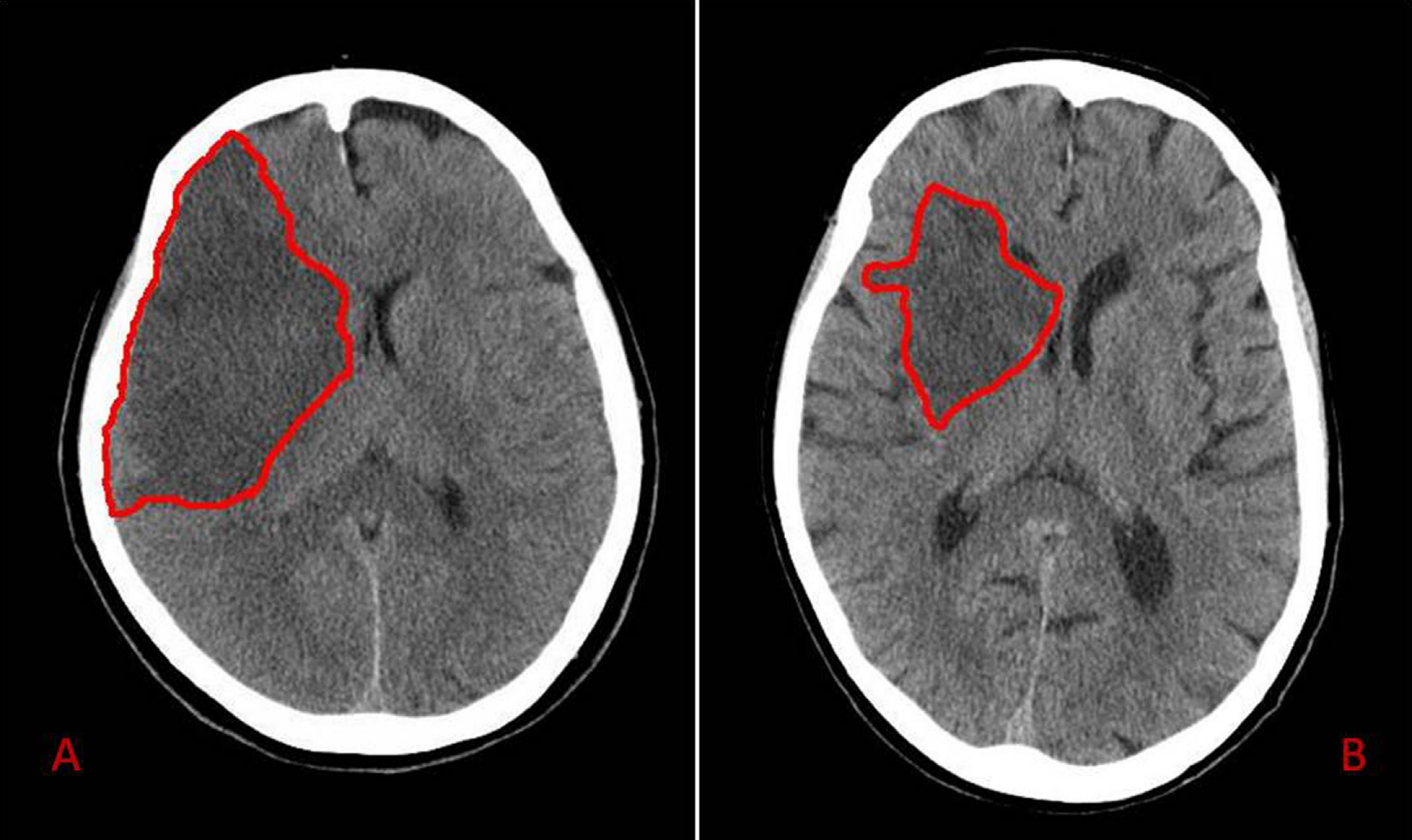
Follow-up brain CT scans of two stroke patients. Two examples of CT scans of two stroke patients at 48 hours since admission. The red outline circumscribes the ischemic area. The patient in panel A had a total occlusion of right middle cerebral artery, presenting with left hemiparesis and hemianopia (Female; Age = 80 yrs.; T0 AEA = 7.31 pmol/lipid mg; T0 NIHSS = 17; T0 aFMS score = 12). The patient in panel B was admitted for a left hemiparesis due to a partial occlusion in right middle cerebral artery territory (Female, Age = 82 yrs.; T0 AEA = 3.93 pmol/lipid mg; T0 NIHSS = 10; T0 aFMS score = 18).
